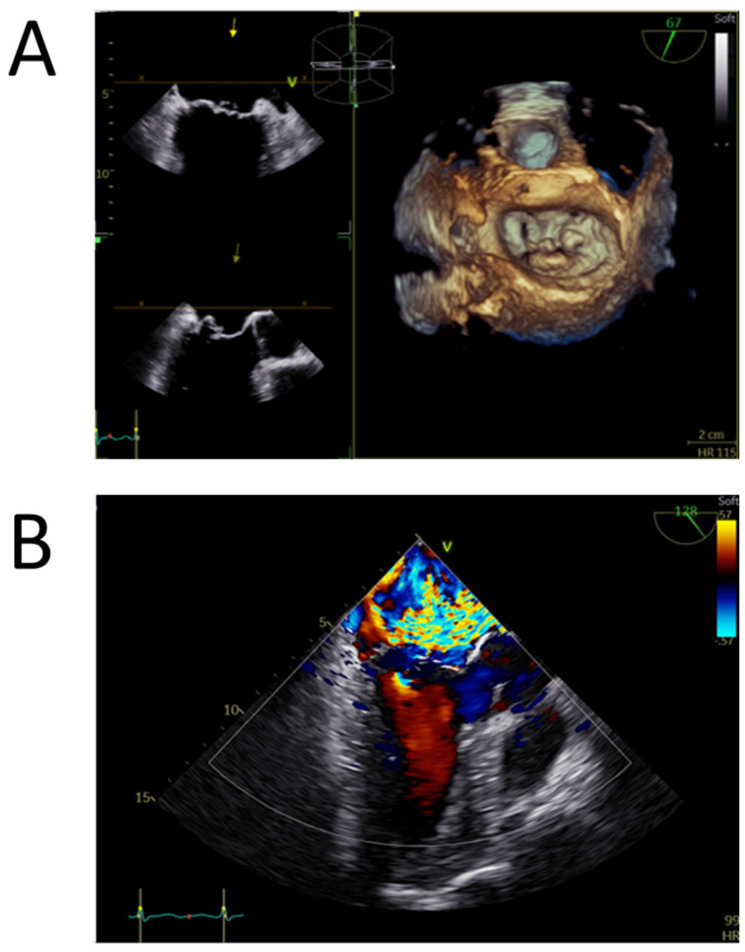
Transoesophageal echocardiography of a myxomatous degenerated mitral valve (Barlow disease). (A) Tridimensional model of the mitral valve positioned in the surgical view obtained by zoom 3D acquisitionextensive multisegmental degeneration, with P2 flail and P1 prolapse. (B) Mid-oesophageal long axis view with color Doppler revealing a wide eccentric, interatrial septum-directed, mitral regurgitant jet, with a large convergence area compatible with severe mitral regurgitation.
